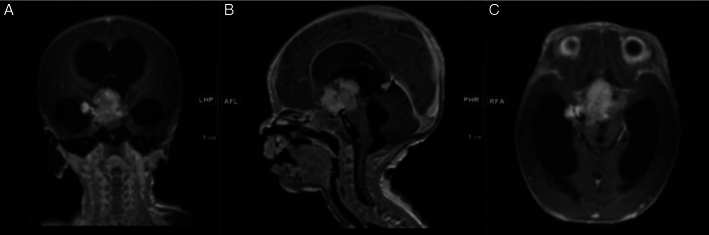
Selected MRI images. T2-weighted MRI head imaging was obtained at the time of our patient’s initial presentation. A, Coronal viewpoint of our patient’s 2.0 ×1.9 ×2.2 cm lesion located along the right suprasellar/interpeduncular cistern. B, Sagittal viewpoint. C, Axial viewpoint with mass effect on the right prechiasmatic optic nerve.
